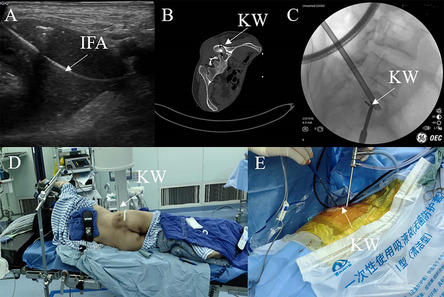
Biportal endoscopic debridement and lavage for sacroiliac joint tuberculosis. (A) Abscess puncture and drainage of anterior iliopsoas muscle guided by B‐ultrasound. (B) Computed tomography‐guided location of sacroiliac joint lesions. (C) The viewing and the working portals for the sacroiliac joint lesions. (D) The patient was placed in a lateral position. (E) Independent viewing and working portals were used to manage the lesions. (IFA, iliac fossa abscess; KW, Kirschner wire.)
